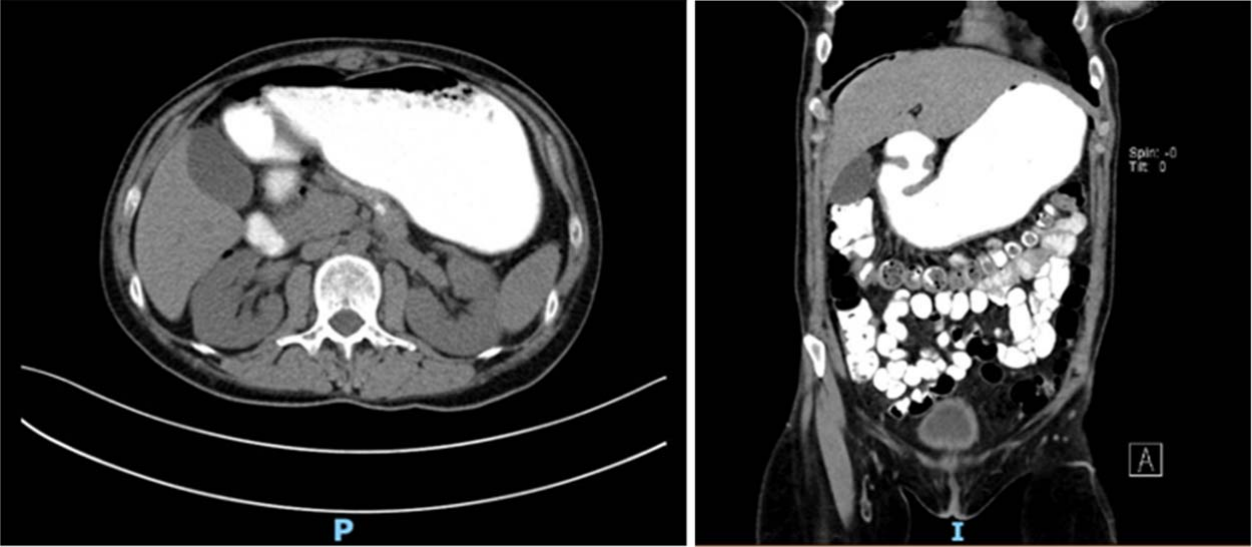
Axial (left) and coronal (right) computed tomography of the abdomen show a distended stomach filled with contrast without any evidence of leaks.
